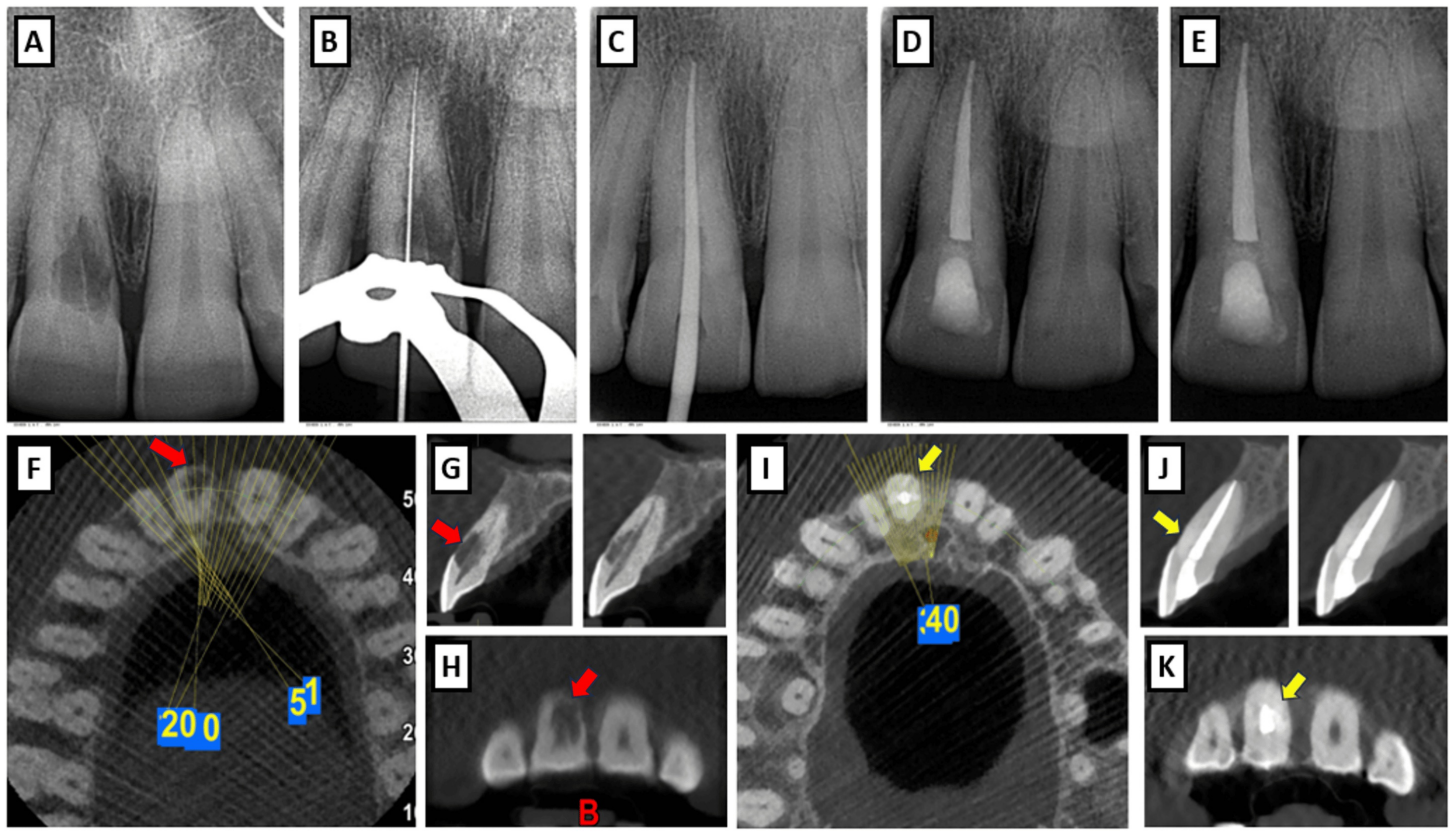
(A) Pre-operative radiograph, (B) Working length radiograph, (C) Master cone radiograph, (D) Immediate post-operative radiograph, (E) One-year follow-up radiograph, (F-H) Pre-operative CBCT images - axial, sagittal, and coronal sections with a slice thickness of 0.5 mm (red arrow denotes the resorption areas), (I-K) Post-operative CBCT images - axial, sagittal, and coronal sections (yellow arrow denotes the intact Biodentine restoration with no further progression of resorption). CBCT, Cone-Beam Computed Tomography
